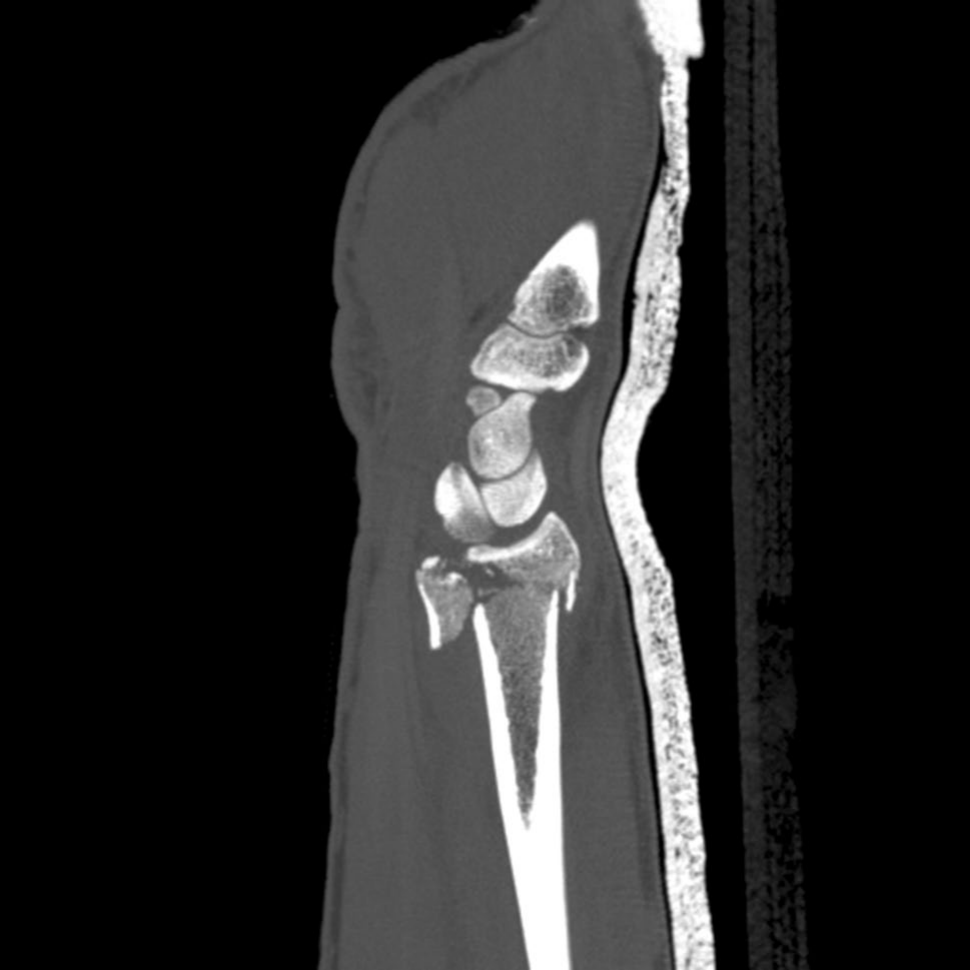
CT scan of a lateral view of the ulnar column showing the additional ulnar fragment flipped 90°
